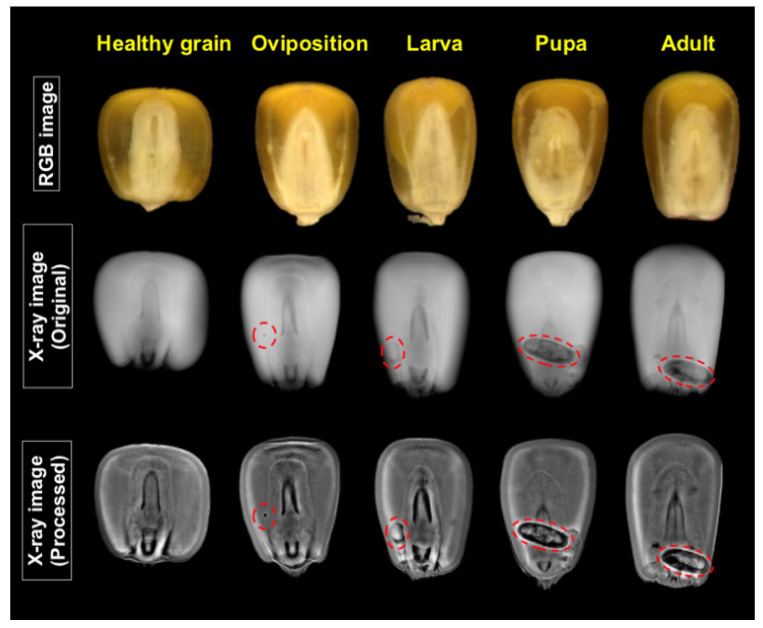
RGB images of non-infested maize grains (healthy grain) and grains infested with *Sitophilus zeamais* during oviposition, larva, pupa and adult stages and corresponding traditional X-ray images and after processing using peripheral equalization and calcification emphasis algorithms. Red circles/ellipses indicate infested regions inside the grain.
